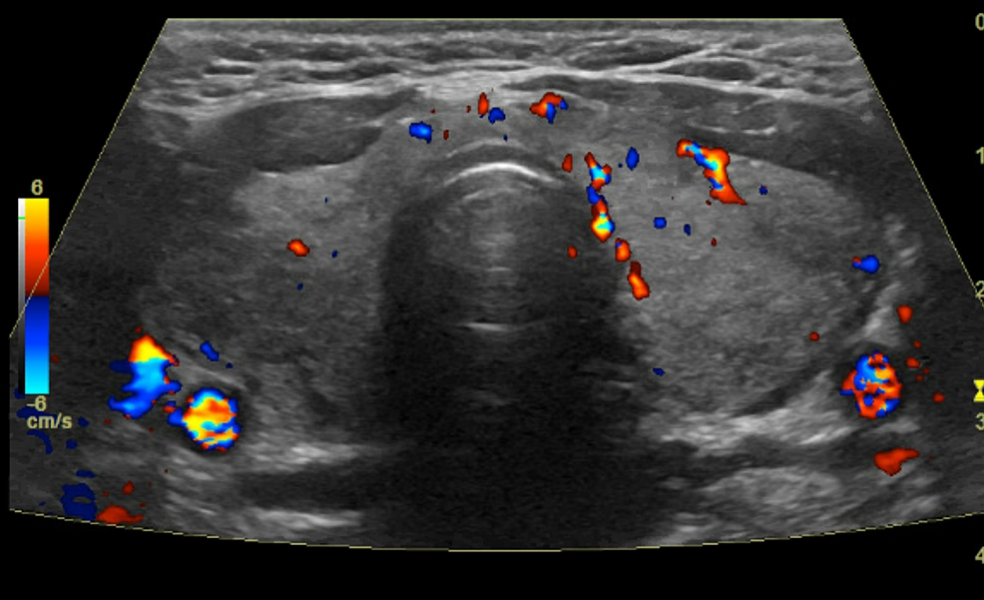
Thyroid ultrasound demonstrating heterogenous echotexture with diminished blood flow on color doppler
